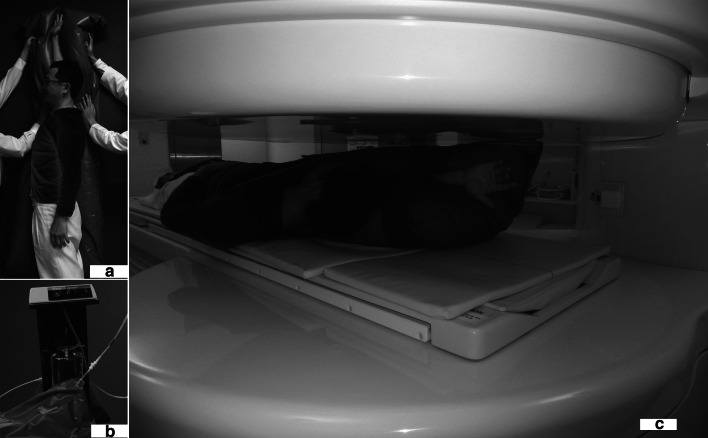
a The arm was placed in maximum elevation in which the tips of fingers reached highest in a standing position. A surgical mattress was adopted around his upper extremity and body to reproduce the position. b The mattress is made of micro-balls of styrofoam, which are stabilized by vacuum. c Volunteer with the mattress, lying in oblique supine position in an open MRI (Magnetom Open, Siemens, Germany)
